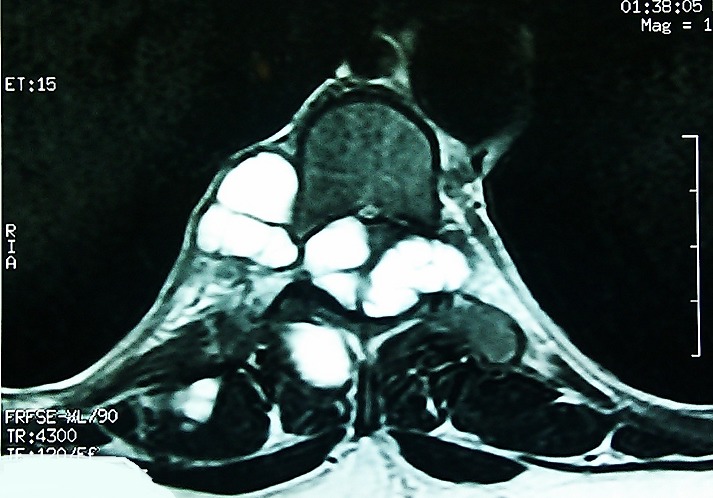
MRI in transversal view showing spinal cord compression by the vesicles of hydatid cysts
